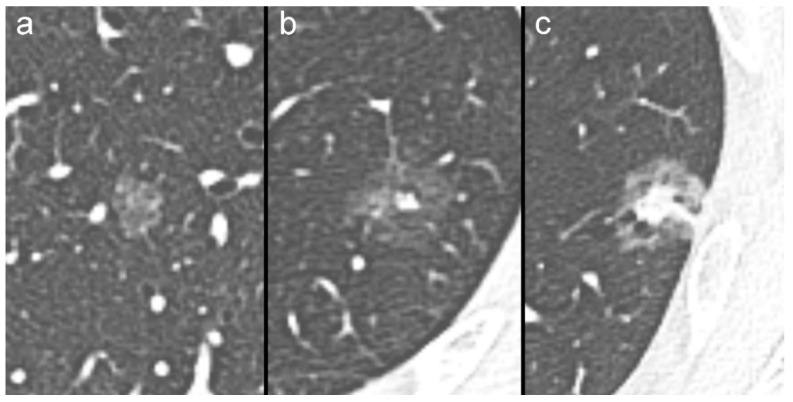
Cropped axial computed tomography (CT) images showing the different subtypes of pulmonary subsolid nodules: (a) non-solid nodule; (b) part-solid nodule with small solid component (less than 6 mm in diameter); (c) part-solid nodule with a large solid component.
